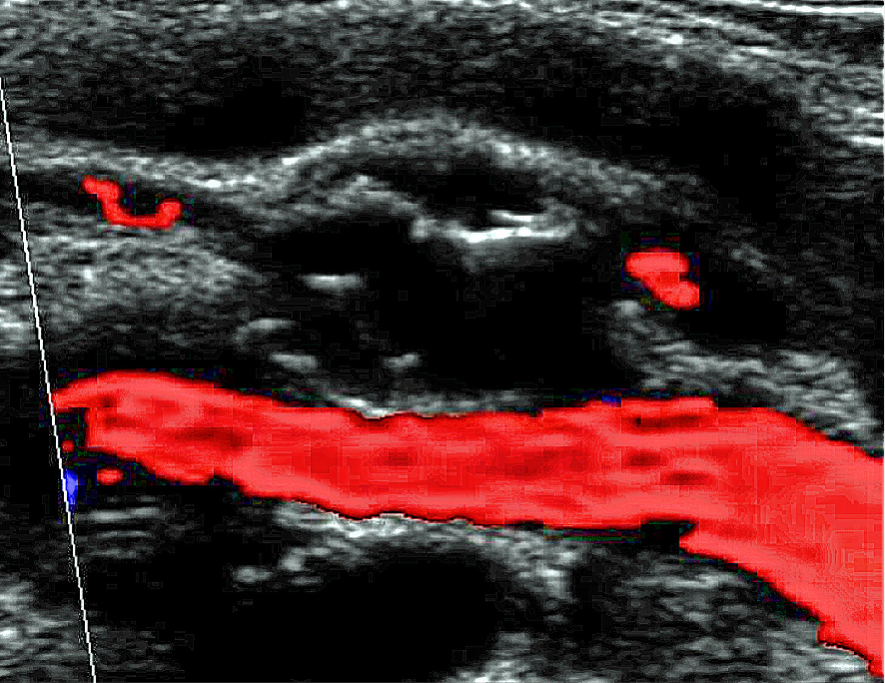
Eco-Doppler colorido arterial demostrando lesão cística septada na camada adventícia da artéria poplítea esquerda, com compressão extrínseca e discreta redução do calibre do vaso.
